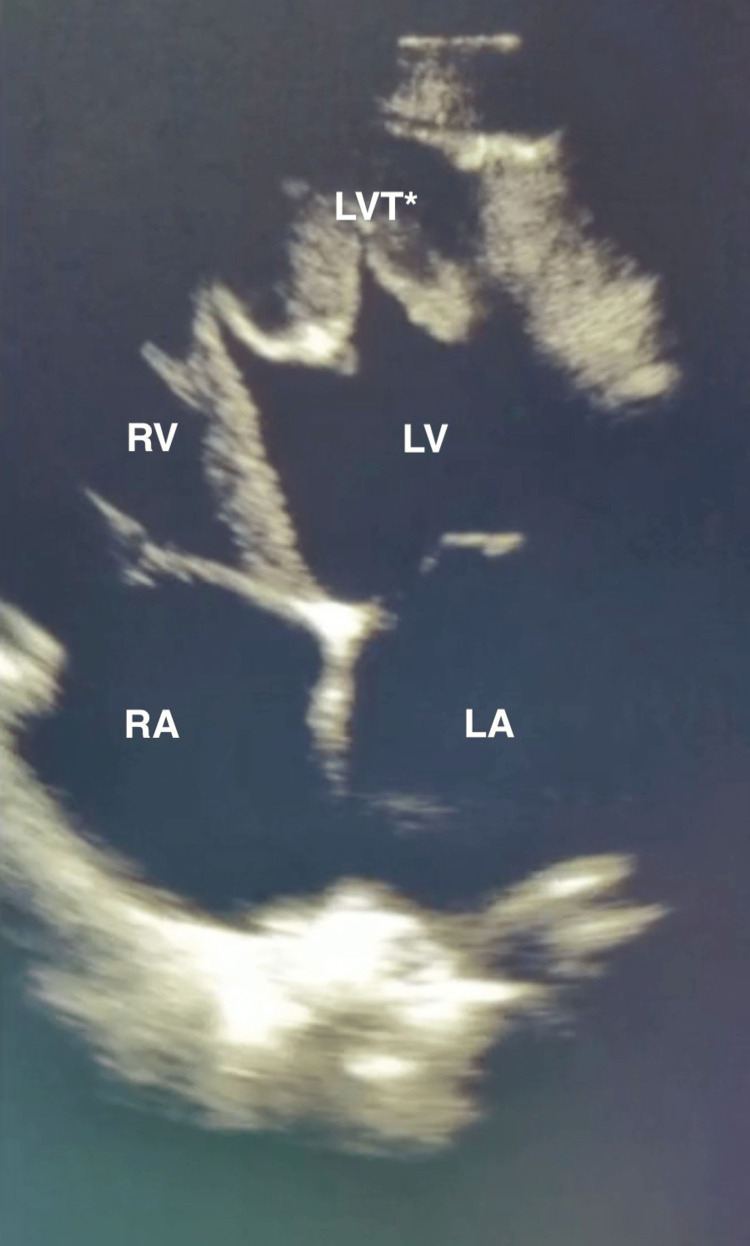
Still image from the bedside transthoracic echocardiogram (apical four-chamber view) that demonstrates a left ventricular thrombus (LVT*) within the left ventricle (LV). Also labeled are the left atrium (LA), right ventricle (RV), and right atrium (RA)
